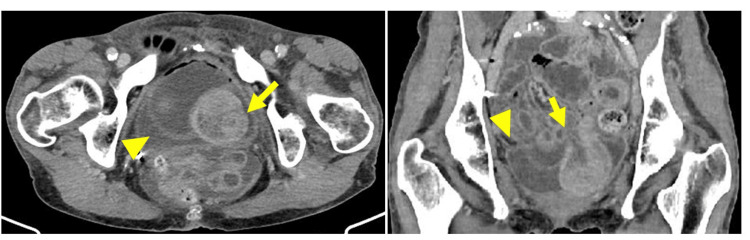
Enhanced CT A: Horizontal: the arrow indicates the small intestine forming loops. The arrowhead points to the bladder; the continuity of the bladder wall appears to be broken. In the horizontal section, it is not clear whether the small intestine is inserted into the bladder. B: Coronal: the small intestine at the arrow forms a closed loop (arrow). The mouth side of the intestine is dilated from there, and it is diagnosed as strangulated ileus. The arrowhead indicates the bladder. Retrospectively, the small intestine is stuck in the ruptured bladder on CT, as seen in the surgical findings. However, it would be difficult to obtain a definitive diagnosis without bladder rupture in mind. CT: computed tomography
